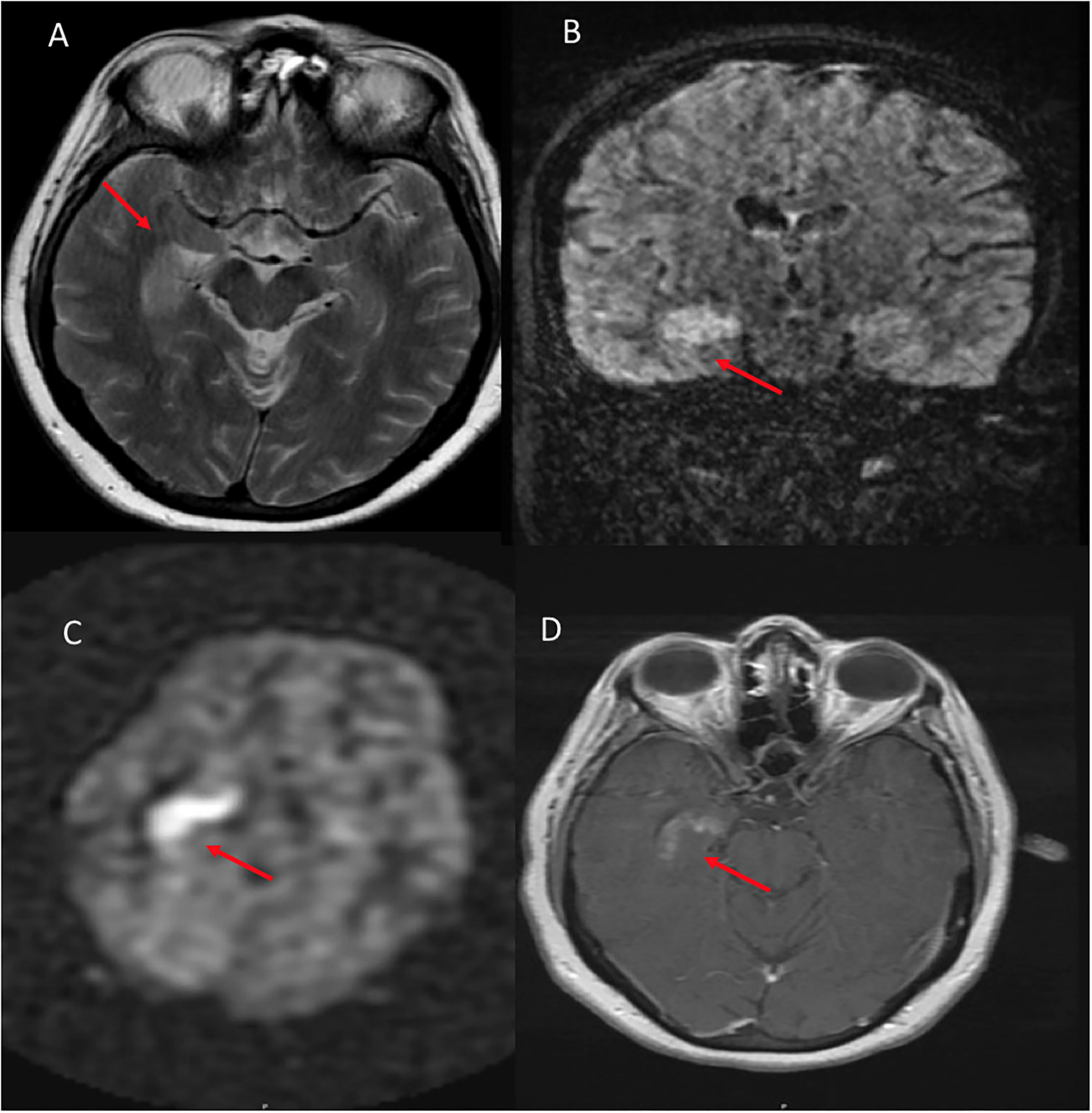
A 54 year old female who presented with altered mental status and seizures. (A) Axial T2W demonstrates right hippocampal hyperintense signal. (B) Coronal FLAIR shows corresponding hyperintense signal in the same region. (C) ASL shows hyperperfusion in the right hippocampus and amygdala. (D) T1W postcontrast shows heterogenous enhancement of the same region.
